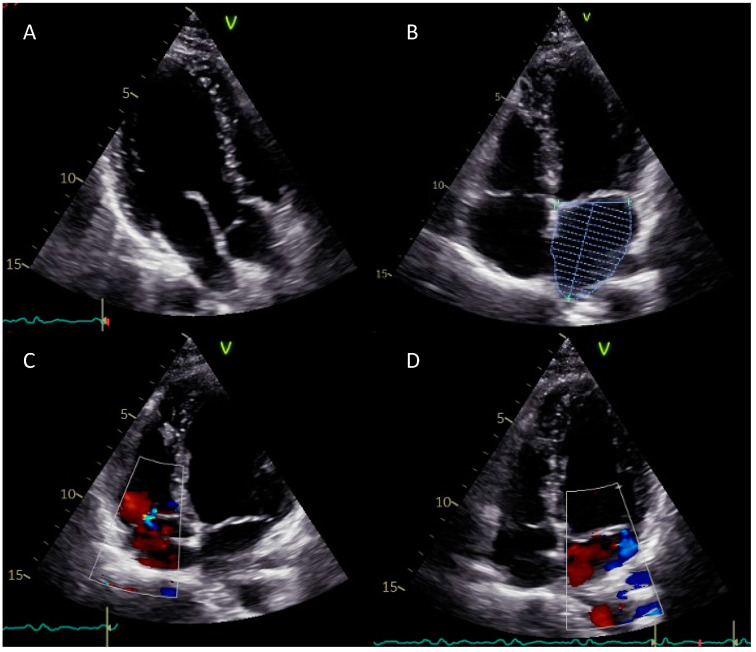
Complete baseline two-dimensional transthoracic echocardiography: three-chamber view (A); four-chamber view (B) with volumetric assessment of the left atrium (32 mL); color-Doppler four-chamber view (C,D). See text above for further details.
